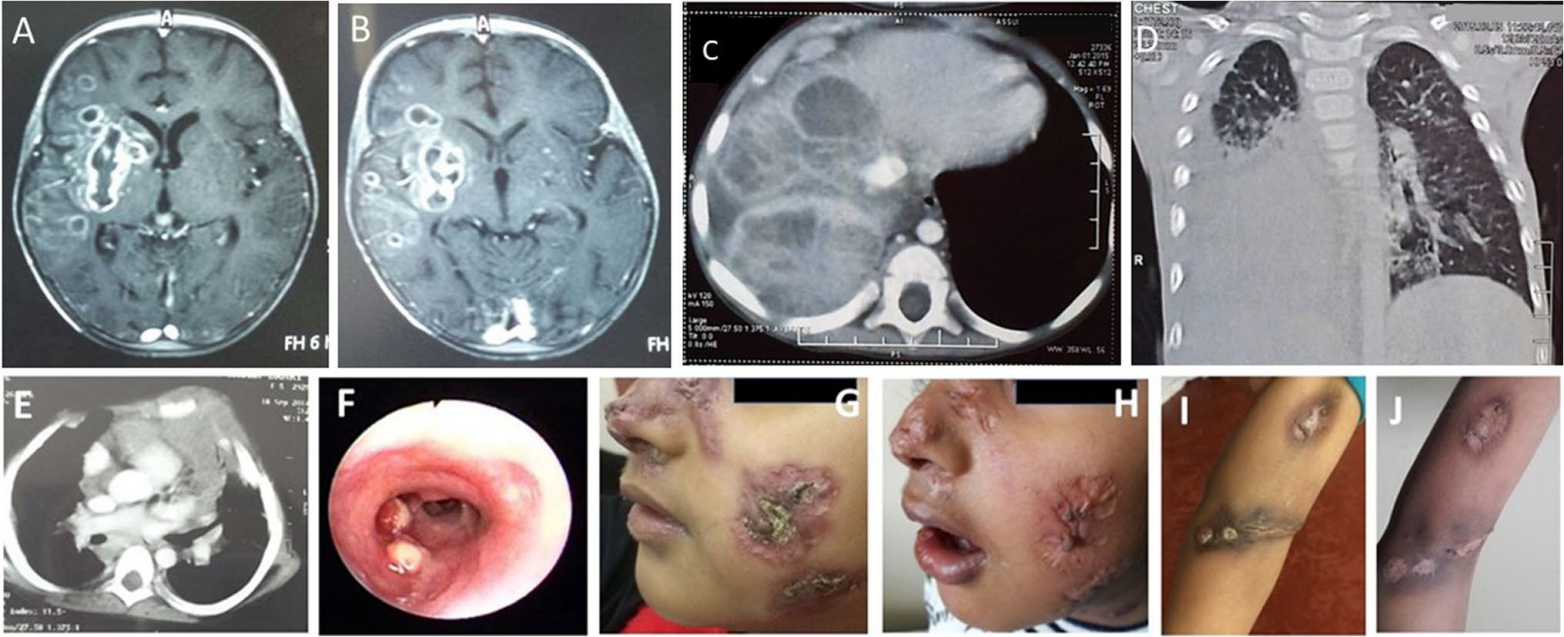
Aspergillus and mycobacterial infections in some patients. A and B, MRI brain T1 post contrast showing right front-temporal marginally enhancing space-occupying lesion; abscess caused by *Aspergillus* infection. C, Post-contrast CT of the abdomen showing right lobe hepatic focal lesion caused by *Aspergillus infection. D*, Shows pulmonary and mediastinal *Aspergillus* infection. E, CT chest showing generalized lymphadenopathy and pulmonary affection due to mycobacterial infection. F, Endoscopy showing bilateral nodular mural lesions in right and left bronchi (atypical mycobacteria). G and I, Mycobacterial skin lesions before treatment. H and J, Mycobacterial skin lesions after anti-tuberculous treatment
